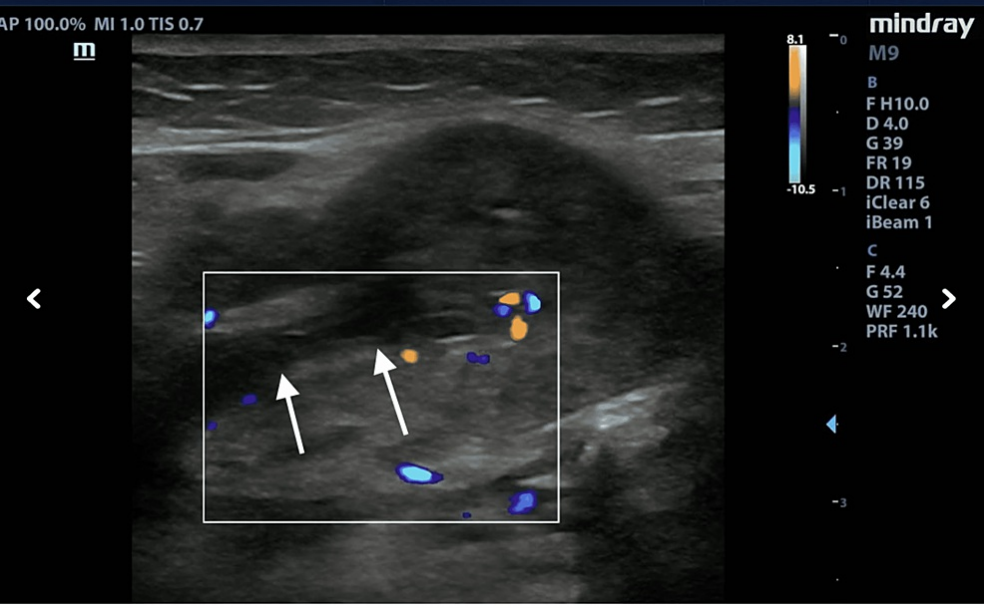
Color Doppler differentiating Wharton’s duct from vascular structures (see arrows).
